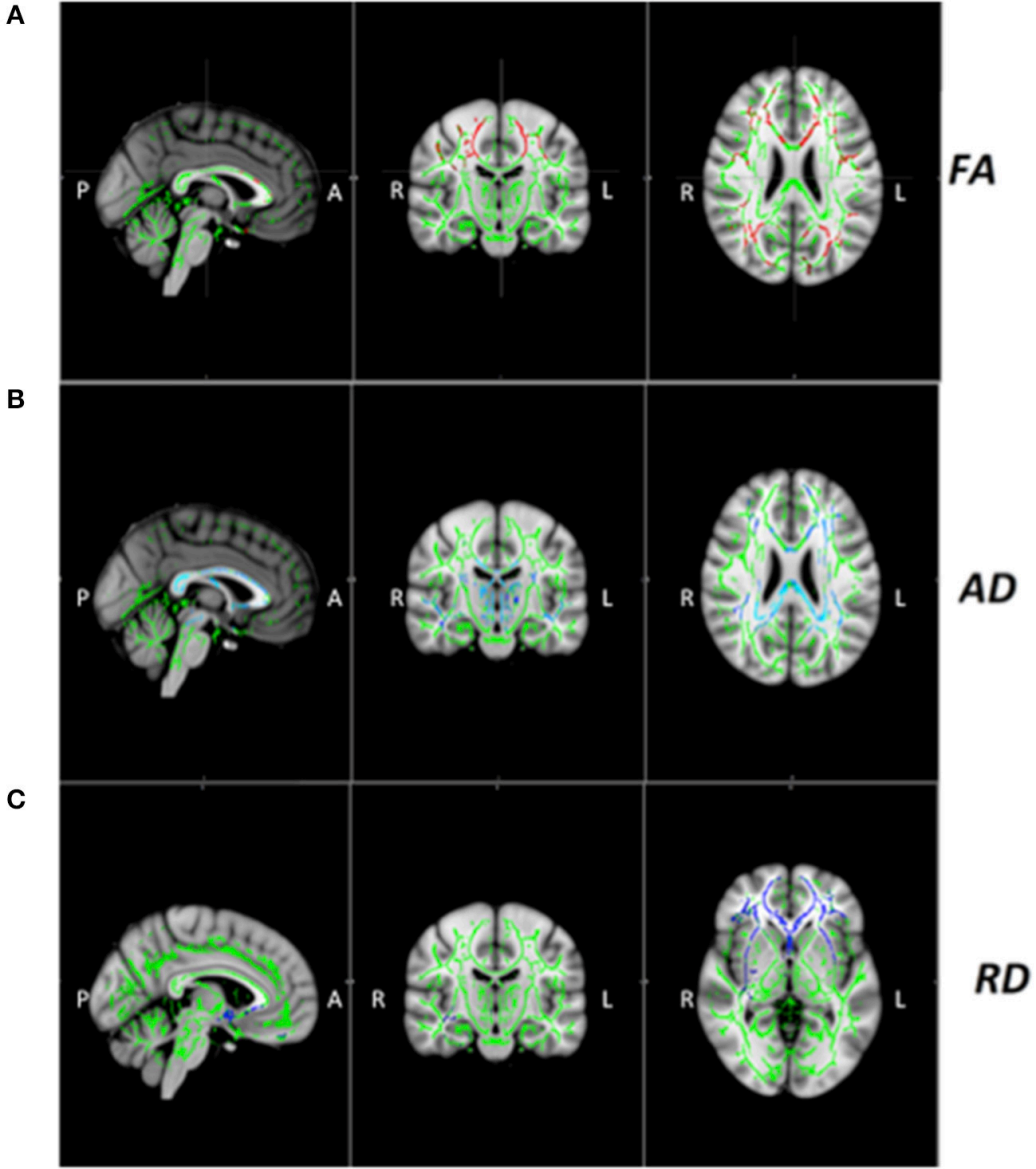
(A-C) White matter diffusion tensor MRI findings in patients with Parkinson's Disease (PD). Decreased fractional anisotropy (FA, red) (*x* = 91, *y* = 109, *z* = 97 MNI coordinates) and increased axial (AD, blue) (x = 93, *y* = 109, *z* = 97) and radial diffusivities (RD, blue) (*x* = 87, *y* = 109, *z* = 66) in patients PD relative to healthy controls (HC).Result are overlaid on the Montreal Neurological Institute standard brain, and displayed at *p* < 0.05 corrected for multiple comparisons at the cluster level using the threshold-free cluster enhancement option. The white matter skeleton is green. L, left; R, right.
